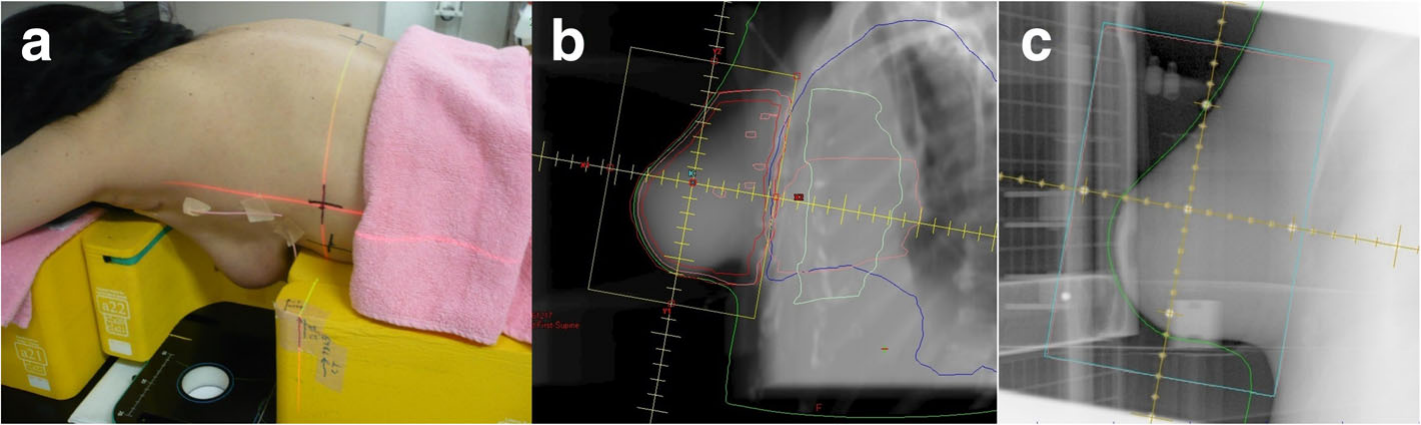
Prone setup of the patient shown in the Fig. 4. a The setup image. b Digitally reconstructed radiography (DRR). c Verification portography
